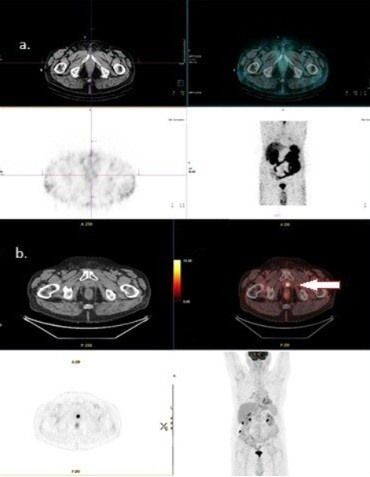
(a) [^99m^Tc]Tc-PSMA is negative in the same patient; (b) [^18^F]FDG PET/CT shows positive findings in the prostatic bed. Original clinical data from University Clinical Center of Serbia, Center of Nuclear Medicine and PET.
